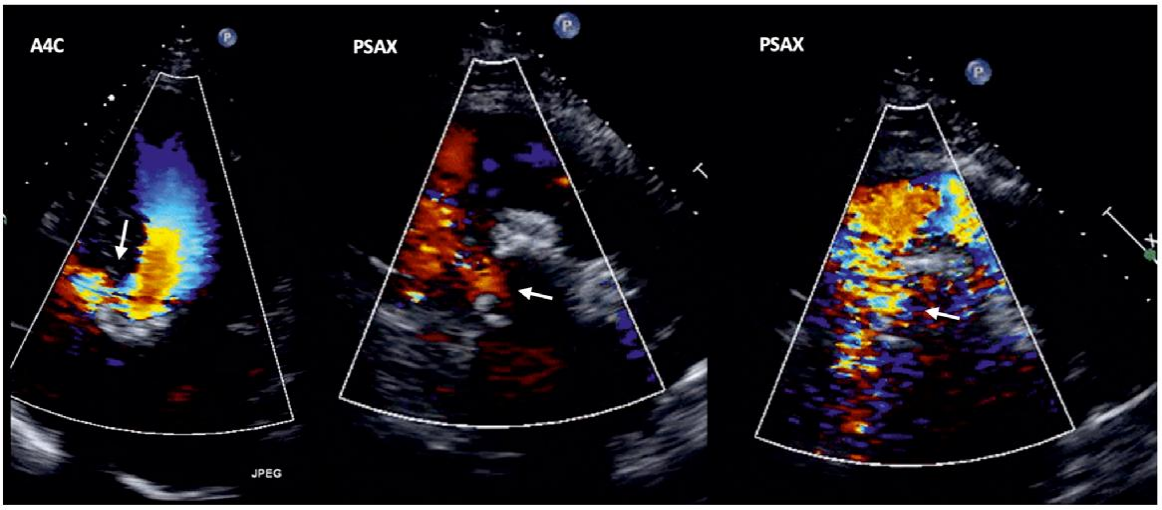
Iatrogenic ventricular septal defect detected by transthoracic echocardiography (Doppler colour). A4C, apical four-chamber; PSAX, parasternal short-axis base.
